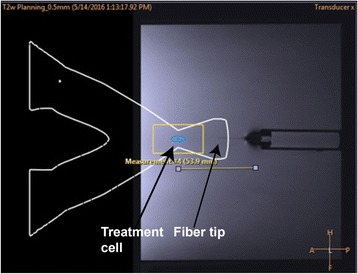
The location of fiber tip inside the water tank in the MR scanner was identified precisely with the help of MR imaging and the HIFU transducer was moved to the corresponding location for the acoustic field calibration
